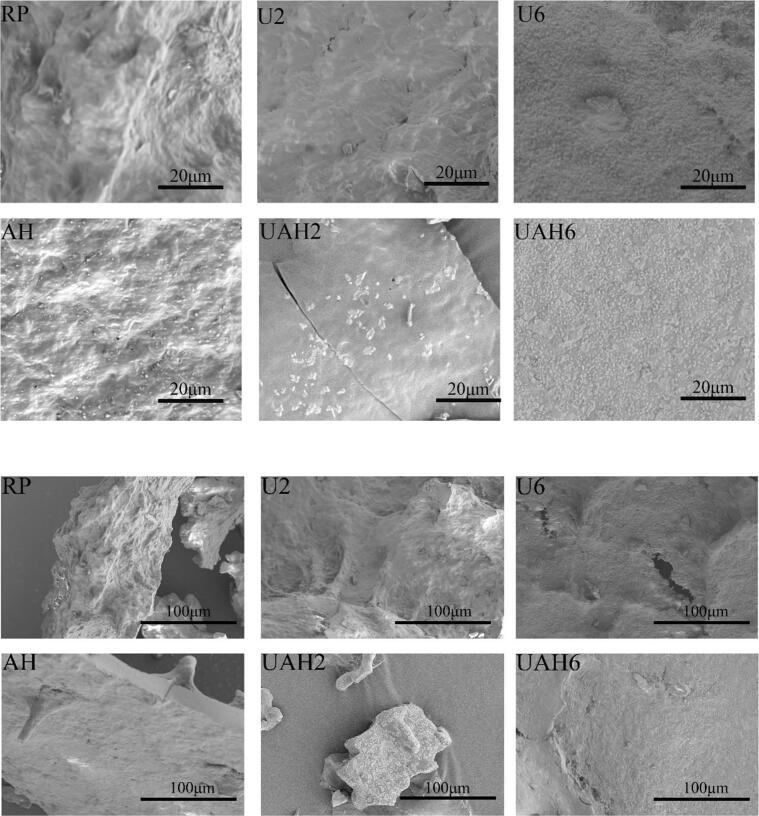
SEM images of different modified rice proteins. Note: Rice protein (RP), Alkali-thermal treatment (AH), 200 W ultrasonic treatment (U2), 600 W ultrasonic treatment (U6), 200 W ultrasonic-alkali-thermal treatment (UAH2), 600 W ultrasonic-alkali-thermal treatment (UAH6).
